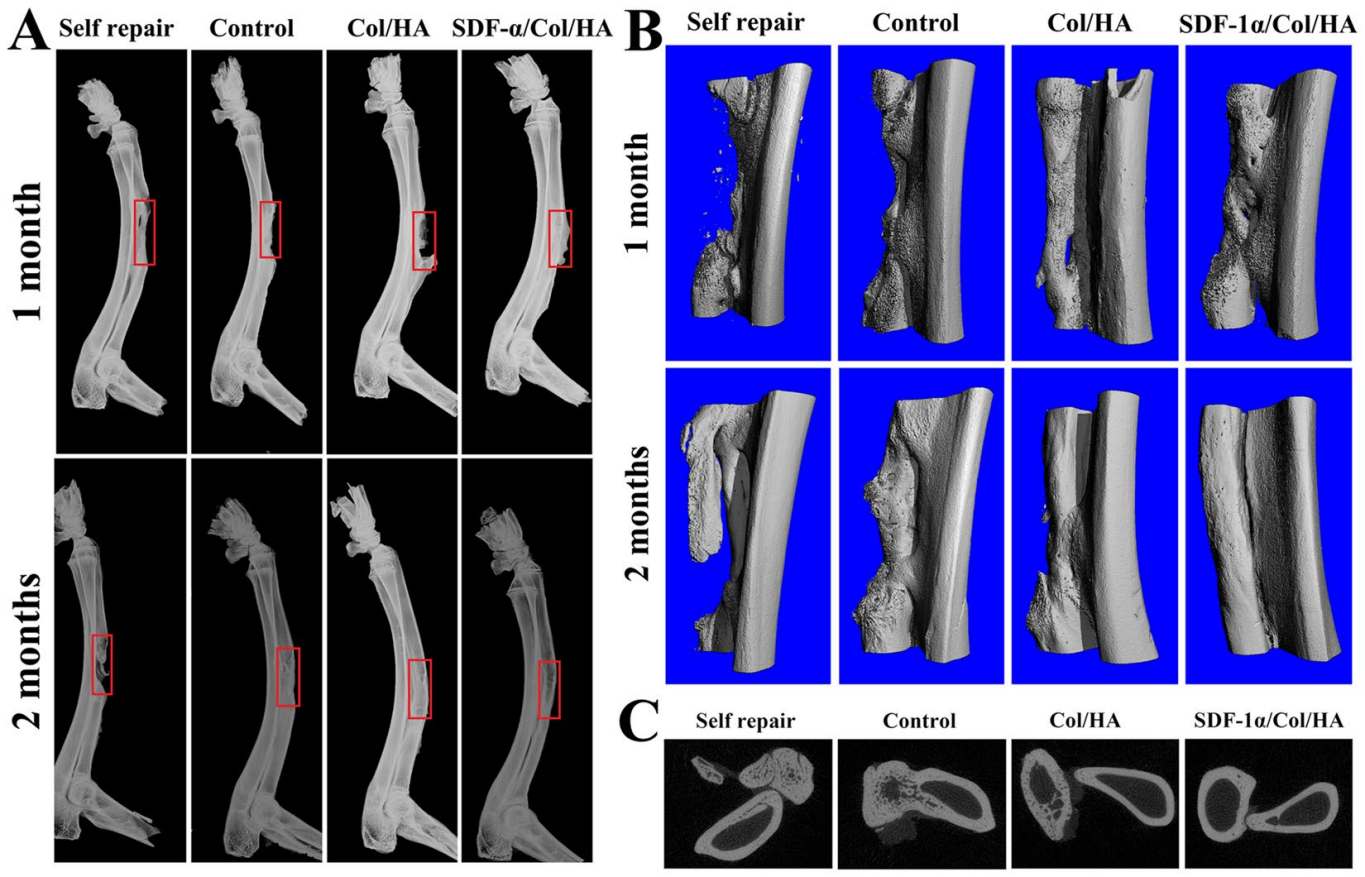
X-ray images (A) and μ-CT 3D images (B) of segmental radius at 1 month and 2 months with different implants. The red wireframe indicates the defective site. 2D section view images (C) of segmental radius at 2 months with different implants.
